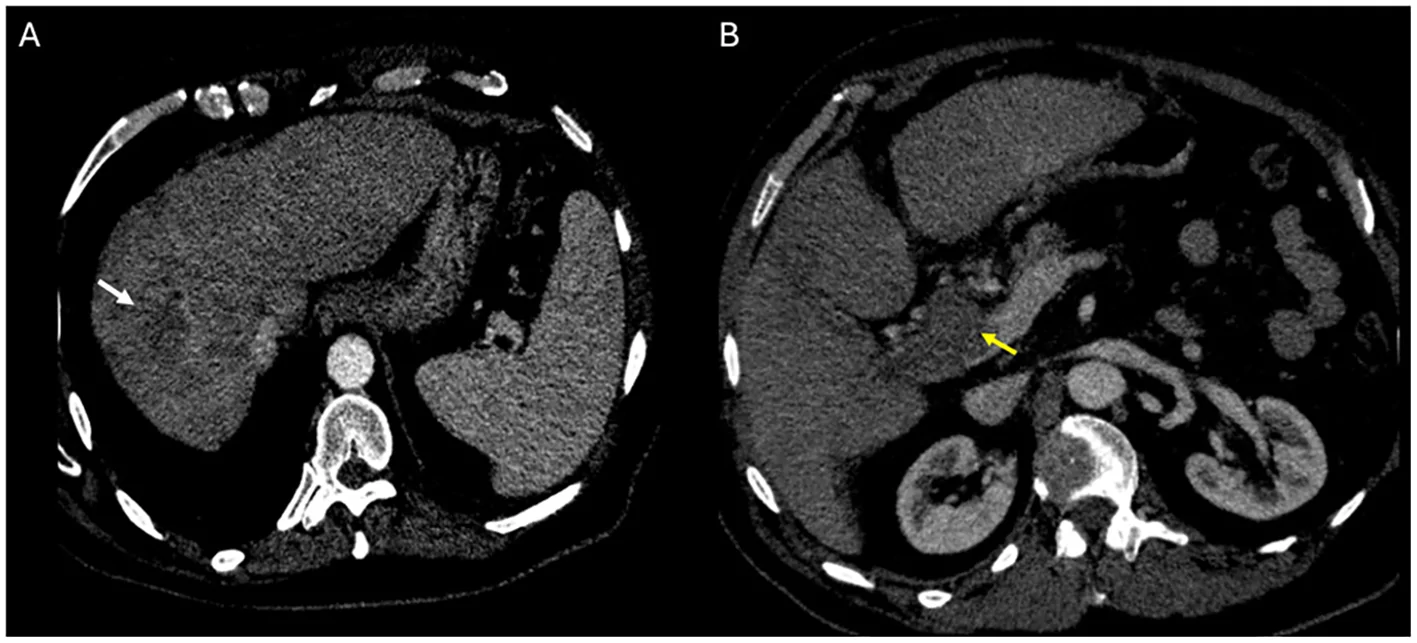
Baseline contrast-enhanced CT (February 2024). (A) Dominant hepatocellular carcinoma involving hepatic segments VII–VIII (white arrow). (B) Extensive portal vein tumor thrombosis (yellow arrow).
